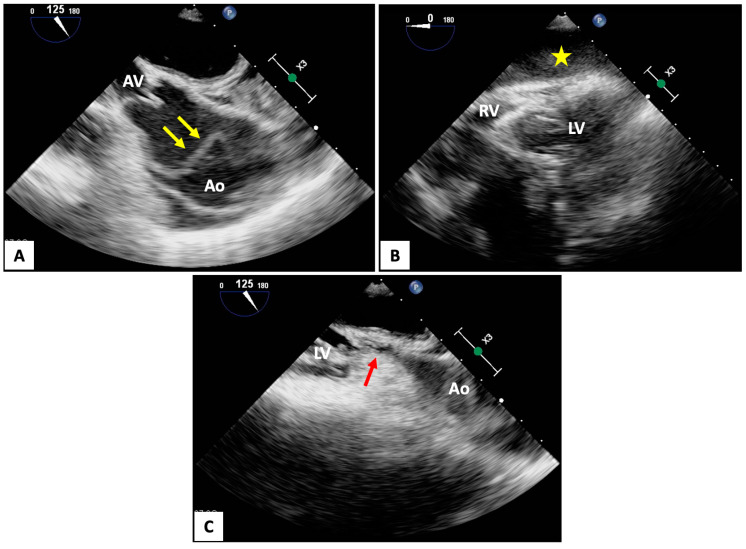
(A,B) Focused transesophageal echocardiography (TEE) images demonstrating a patient with a type A aortic dissection (yellow arrows) complicated by hemopericardium with tamponade (yellow star). (C) The area of maximal compression can be seen at the aortic root (red arrow). Ao, aorta; AV, aortic valve; LV, left ventricle; RV, right ventricle.
